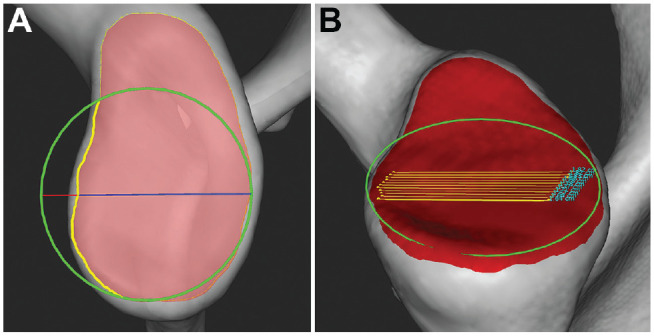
Evaluation of a shoulder CT image using ImFusion software. (A) Linear- and area-based glenoid bone loss were calculated by projecting the glenoid mesh contour (red shaded area) onto the BFC plane (green circle). Linear-based glenoid bone loss was defined as the maximum perpendicular distance between the BFC and the glenoid contour projection (red line), and area-based glenoid bone loss was defined as the BFC area minus the area of the glenoid contour projection inside the BFC (yellow line). (B) To compute the BSSR, the concavity depth was determined. For this purpose, the glenoid mesh was cut along its short axis by a plane passing through the BFC center, and the concavity depth was estimated as the farthest point from a line parallel to the glenoid short axis that touched the glenoid mesh. As the measurement is very sensitive to glenoid mesh irregularities, the calculation was repeated along 10 different mesh cuts, performed with planes parallel to the first plane (yellow lines), to produce the final concavity depth value as an average.
